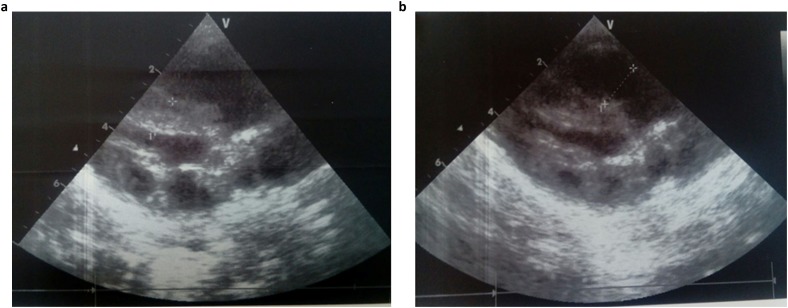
Echocardiogram at the age of 5 months showing concentric HCM (a) and right ventricular outflow tract dilation (b).
